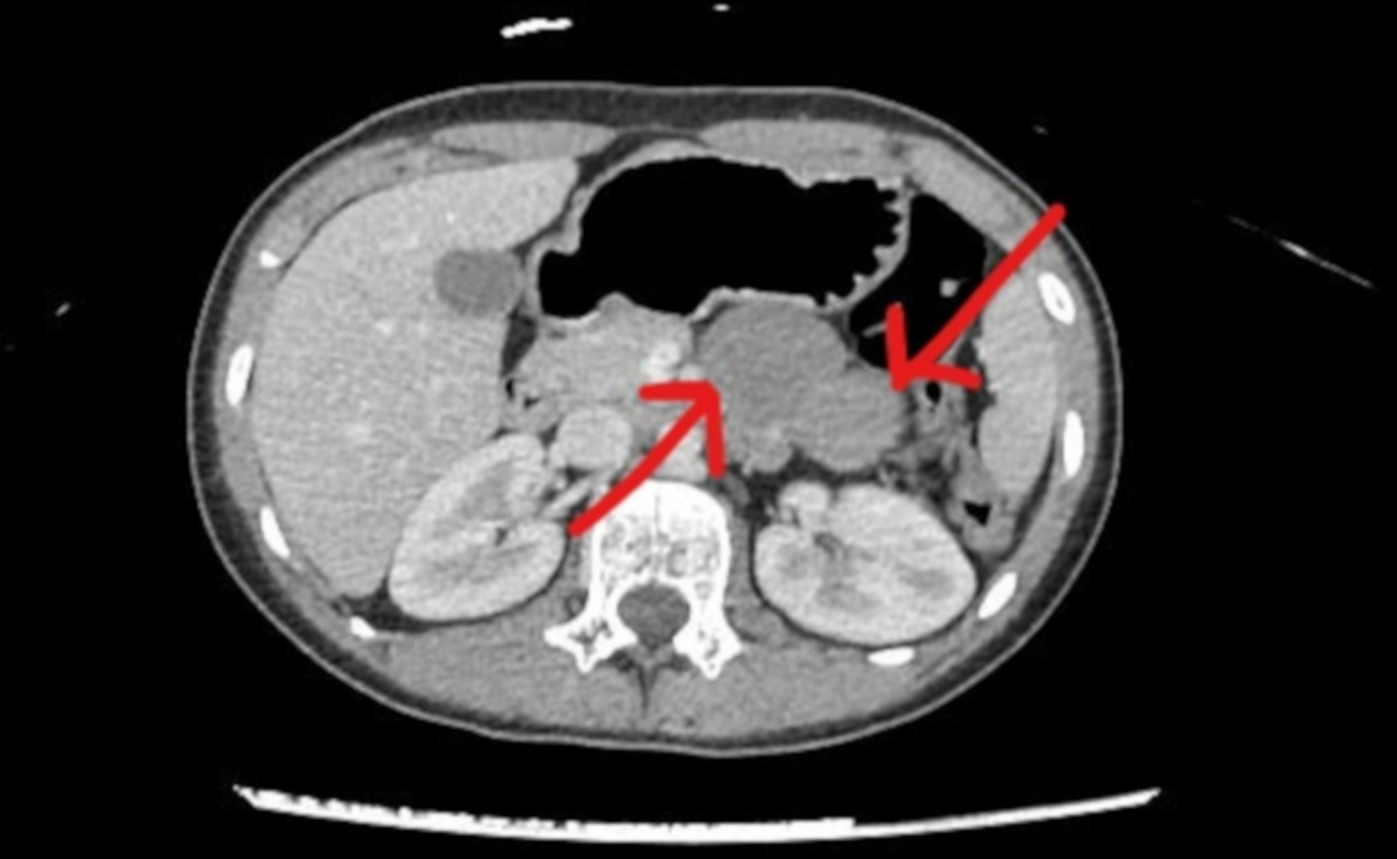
Computed tomography (CT) scan of the abdomen, with contrast, showing a bi-lobed soft tissue density lesion (arrows), inseparable from the pancreas
